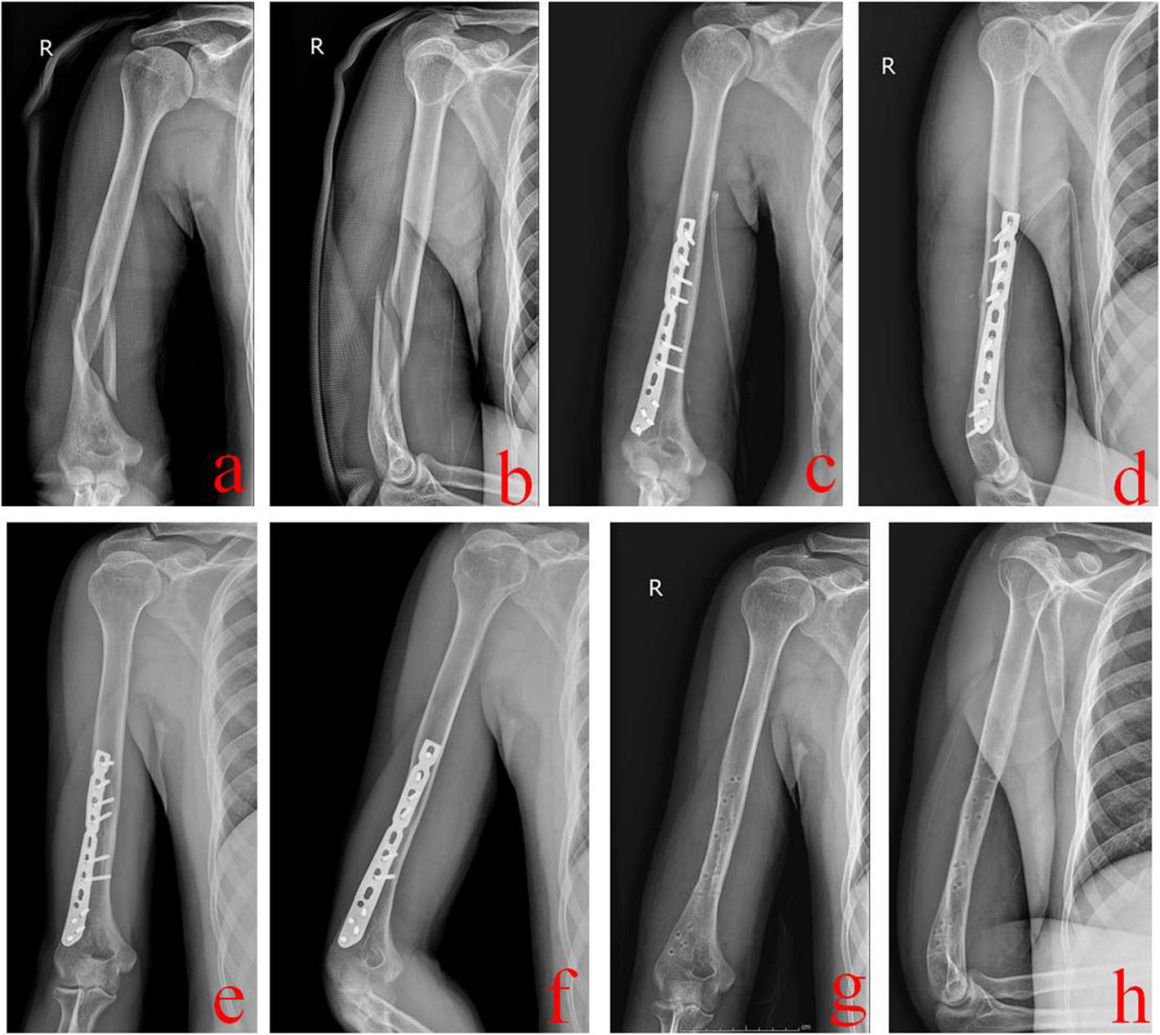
A 24-year-old male was treated by AATI technique. a and b Preoperative X-ray films showed a middle and distal-third humeral shaft fracture. c and d Immediate postoperative X-ray films showed good reduction and fixation. e and f One year after operation, X-ray films showed that this fracture healed well. g and h X-ray films after removal of the internal fixation plate. AATI stands for the anterolateral approach with two incisions
